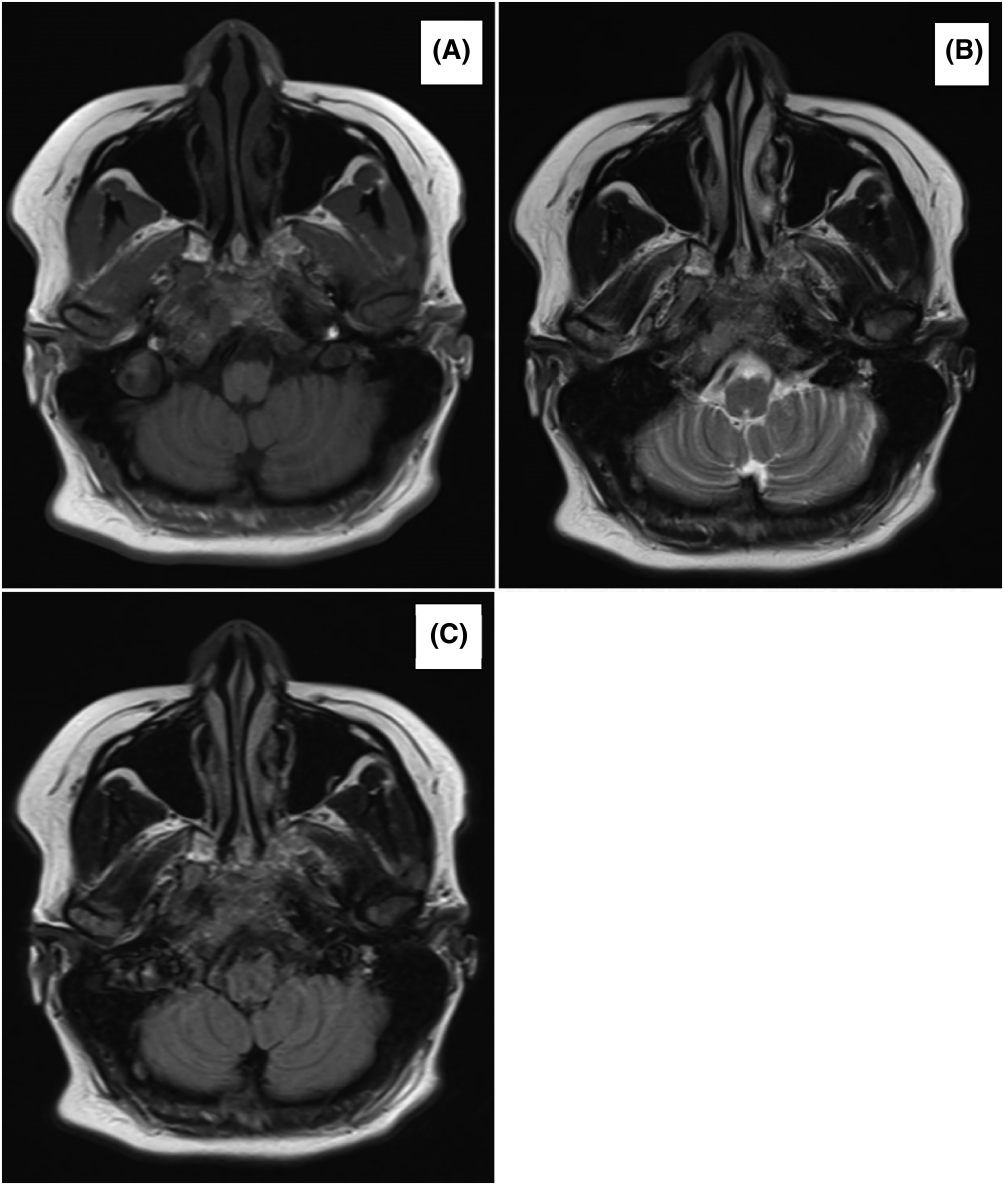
A magnetic resonance image (MRI) of the brain revealed marked heterogeneity of marrow signal, involving clivus and extends to petrocival bilaterally, sphenoid and abutting the cavernous sinus. (A) T1; (B) T2; (C) T1 post contrast.
